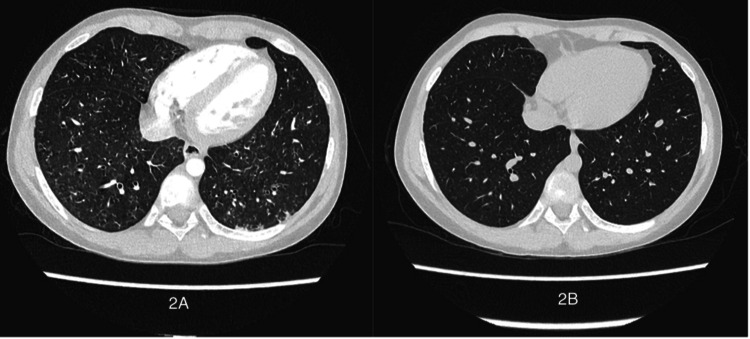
(2-A) Chest CT before treatment with inhaled corticosteroids, showing diffuse centrilobular nodular infiltrates and bilateral peribronchial thickening, with no evidence of bronchiectasis or interstitial ground-glass infiltrates. (2-B) Chest CT after treatment, showing complete resolution of the abnormal infiltrates.
